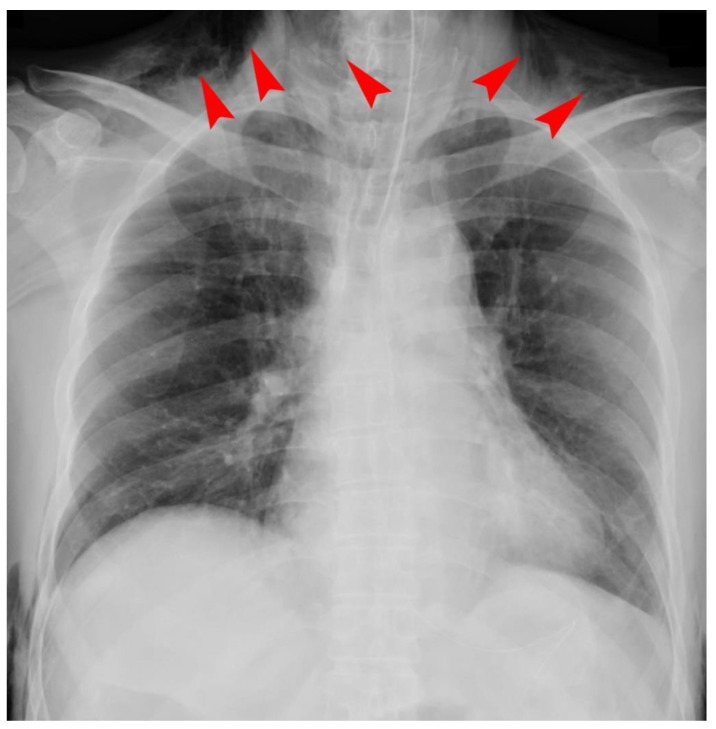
A chest X-ray of a serious case of subcutaneous emphysema. Subcutaneous emphysema (SCE) massively extended as far as the neck region in 12 serious cases among 55 SCE (+) cases. Arrowheads indicate SCE in the neck region.
